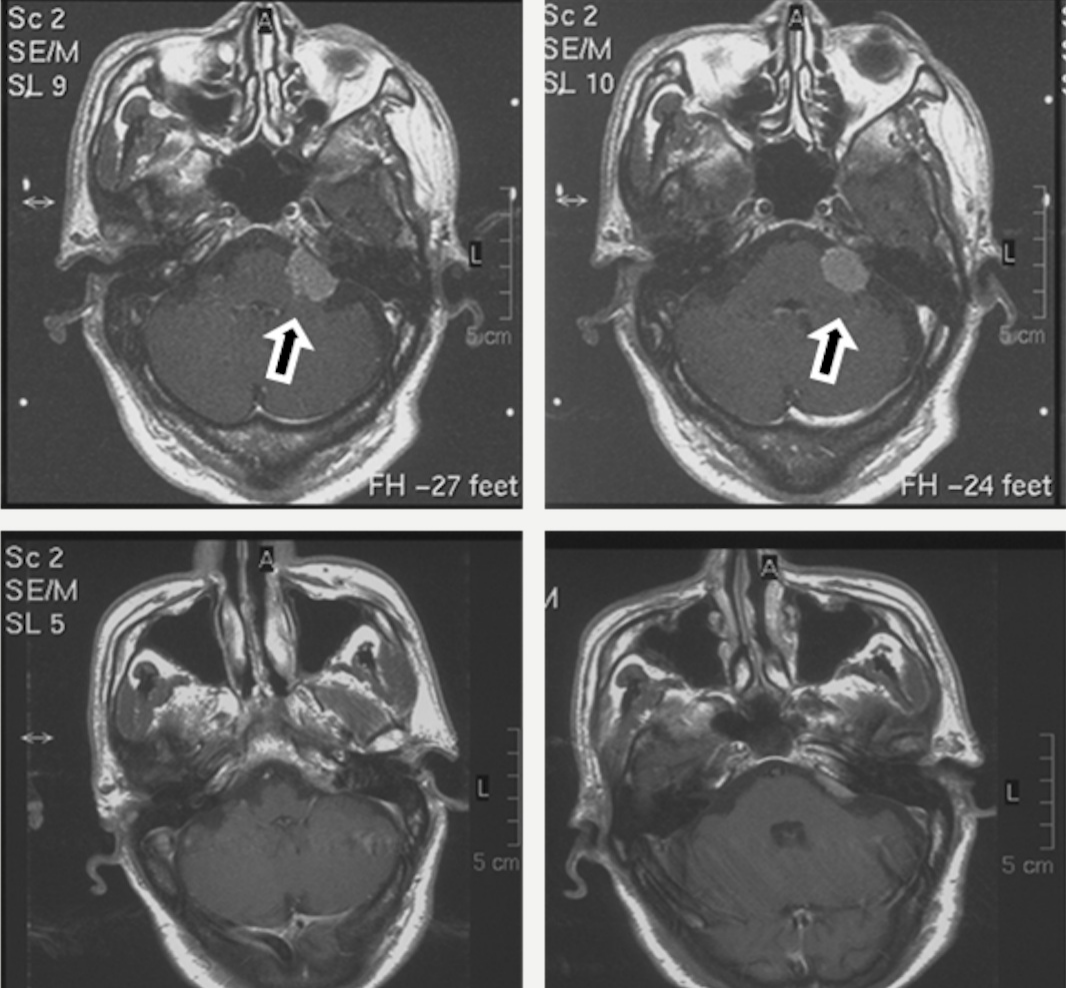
Application of Radiosurgery to Residual Tumor MRI with contrast of postoperative radiotherapy for the residual tumor. The residual of the CPA meningioma (upper row, arrow) underwent 16 Gy stereotactic radiosurgery 30 days after operation, and it disappeared two years later (lower row). CPA: cerebellopontine angle; MRI: magnetic resonance imaging
